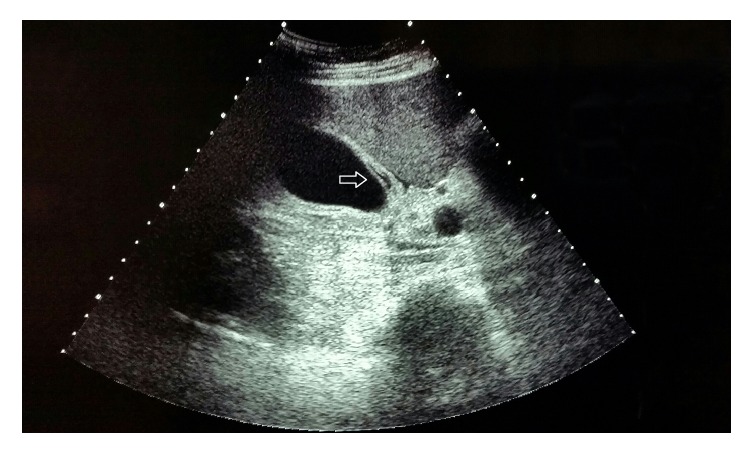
Ultrasound of the gallbladder, revealing thickened gallbladder wall with a layered appearance (arrow).
